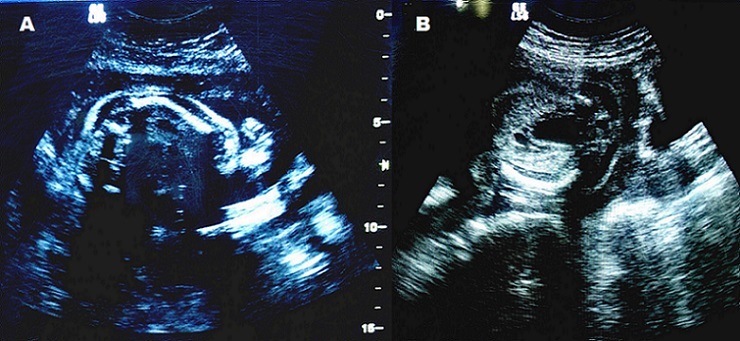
Prenatal ultrasonography at 18 weeks of gestation: (A) curved right femur; (B) curved left femur
